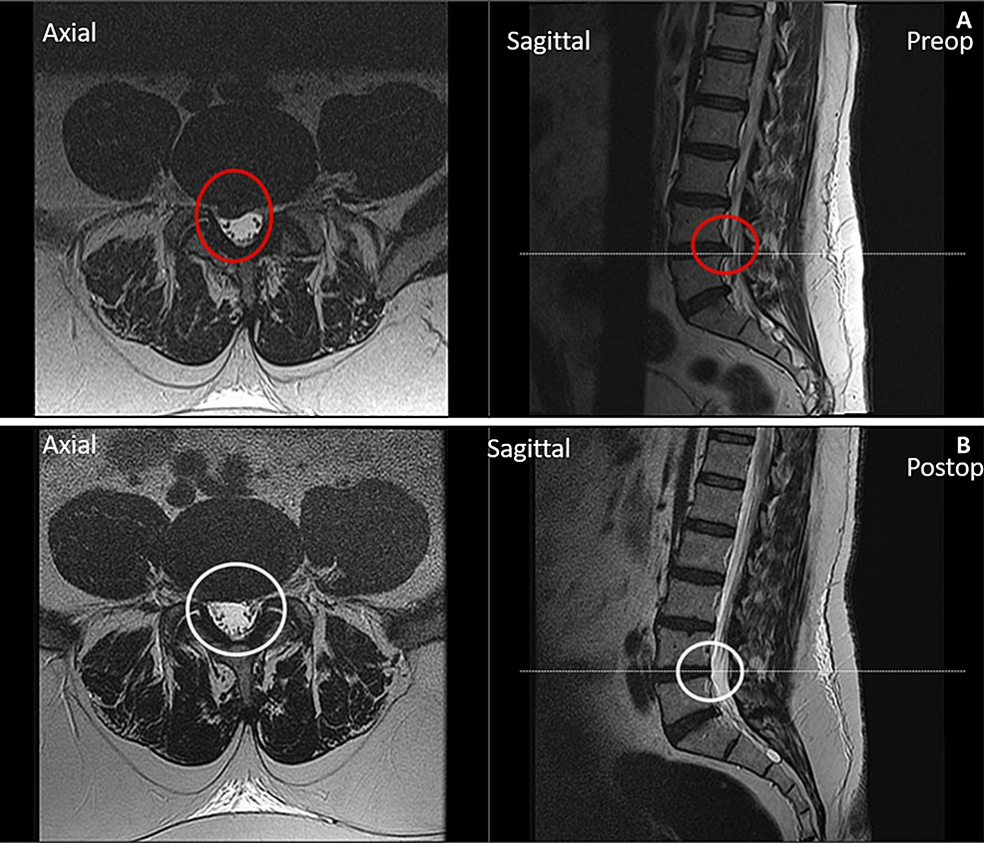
Magnetic resonance imaging (MRI) of the lumbar spine (A) The preoperative MRI axial (left) image of the lumbar spine at the L4-5 level, as indicated by the dashed line on the sagittal (right) image. The red circles highlight the pathology, namely a left-sided disc protrusion with impingement of the thecal sac. (B) The postoperative MRI axial (left) image of the lumbar spine at the L4-5 level is indicated by the dashed line on the sagittal (right) image. The white circles reflect the surgical correction of the pathology presented in panel A.
